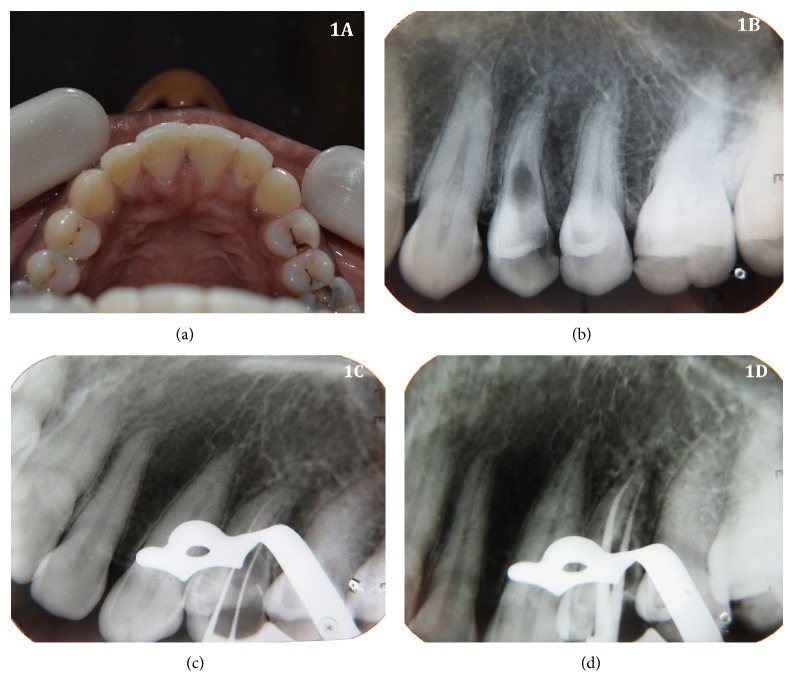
(a) Clinical photograph of maxillary arch occlusal view. (b) Preoperative radiograph. (c) Working length determination radiograph. (d) Master cone selection radiograph.
